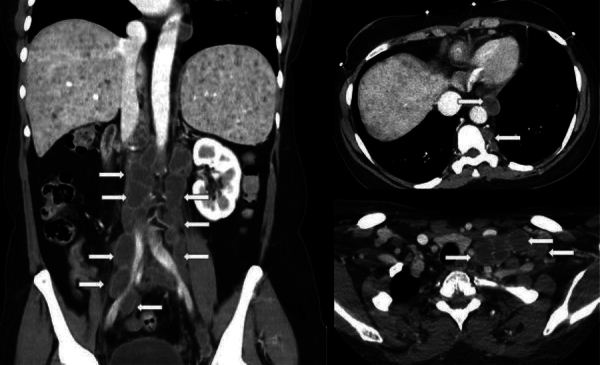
Coronal and axial contrast-enhanced CT
